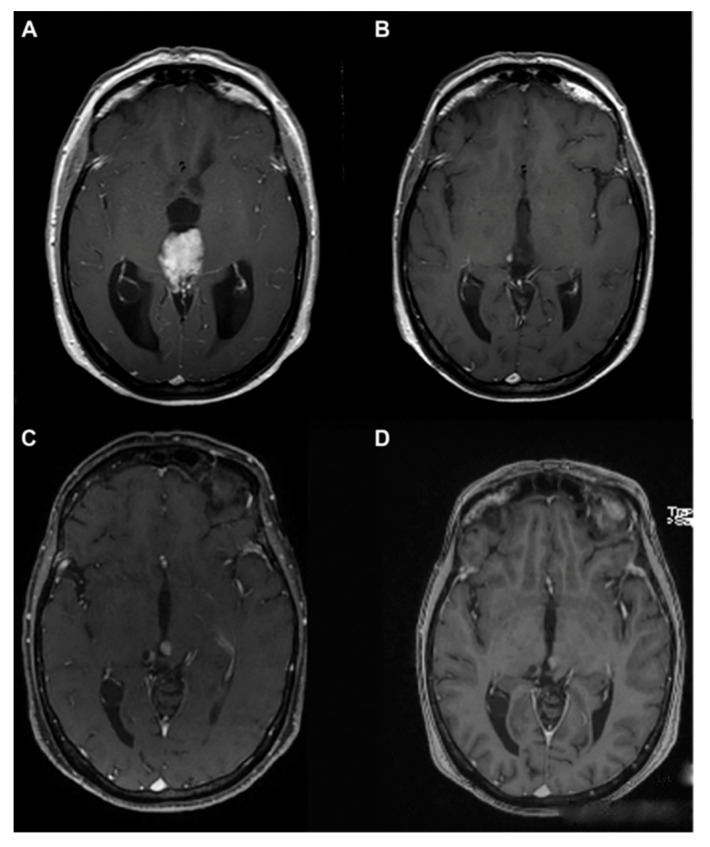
Pineal parenchymal tumors of intermediate differentiation MRI. Preoperative MRI (A), postoperative MRI showing a very small residual pineal lesion (B). MRI before (C) and after (D) radiation therapy. Reprinted with permission from Choque- Velasquez et al. (2019) [102]. Elsevier—2021 (License Number: 5035440109275). Pineal parenchymal tumors of intermediate differentiation may be morphologically classified into (1) lobulated endocrine-like and highly vascular lesions; (2) those with diffuse growth patterns, similar to oligodendrogliomaneurocytomas; and (3) those of transitional type, with areas of lobulated and diffuse growth patterns, correlated with areas of pineocytomatous rosettes [82,102,109]. Recently, Wu et al. [103] reported that CD24 and PRAME may be novel markers useful for the grading and prognostic evaluation of pineal parenchymal tumors of intermediate differentiation and, thus, useful in therapeutic decision-making. The best treatment for pineal parenchymal tumors of intermediate differentiation has not yet been found, partly due to the low numbers of reported cases. The maximal surgical removal of the tumor is the optimal treatment in practice for pineal parenchymal tumors of intermediate differentiation. However, even after complete surgical tumor removal, many patients experience recurrence. Hence, adjuvant radio- or chemo-therapy, or a mix of both, is often recommended to improve patient survival. A report from the International Gamma Knife Research Foundation described actuarial local control and survival rates following stereotactic radiosurgery on pineal parenchymal tumors of intermediate differentiation of 50% and 56%, respectively, at 5 years [19,26].
